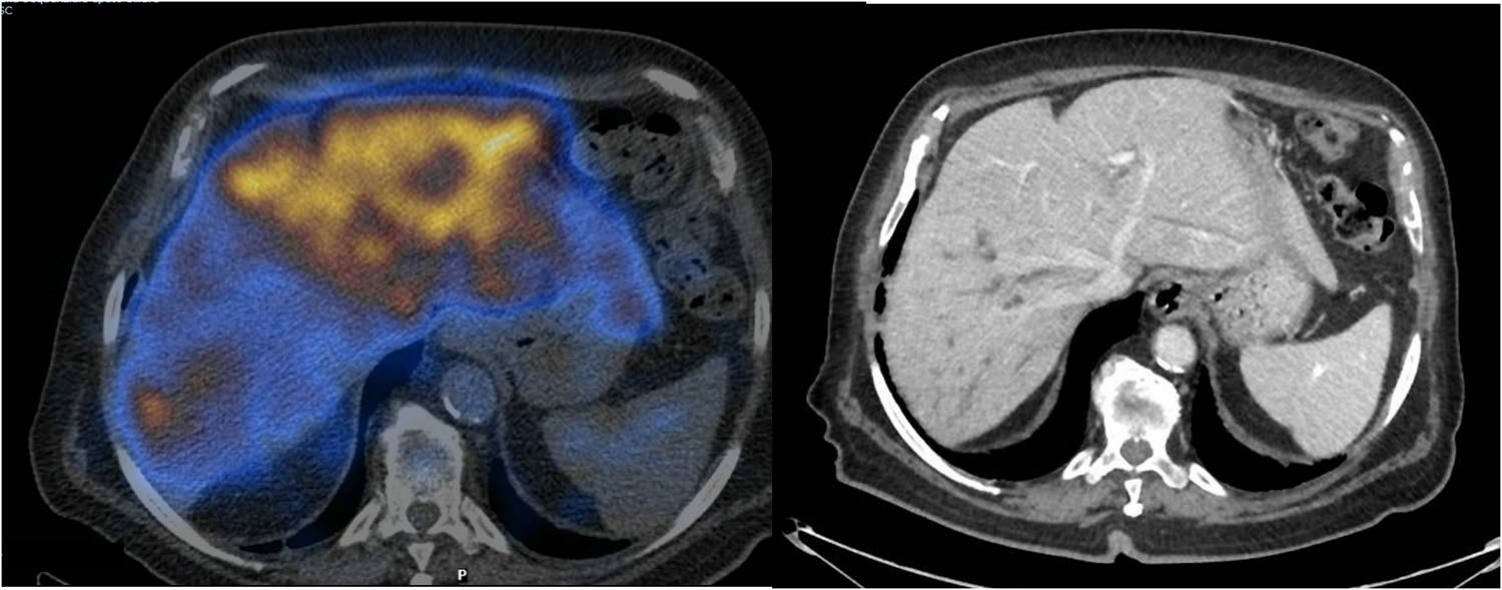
SPECT showing the distribution of function within the liver (a). The tumor, occupying entirely the right lobe, transferred the liver function almost to the left side (i.e. the future liver remnant). Abdominal computed tomography of the same patient (b)
